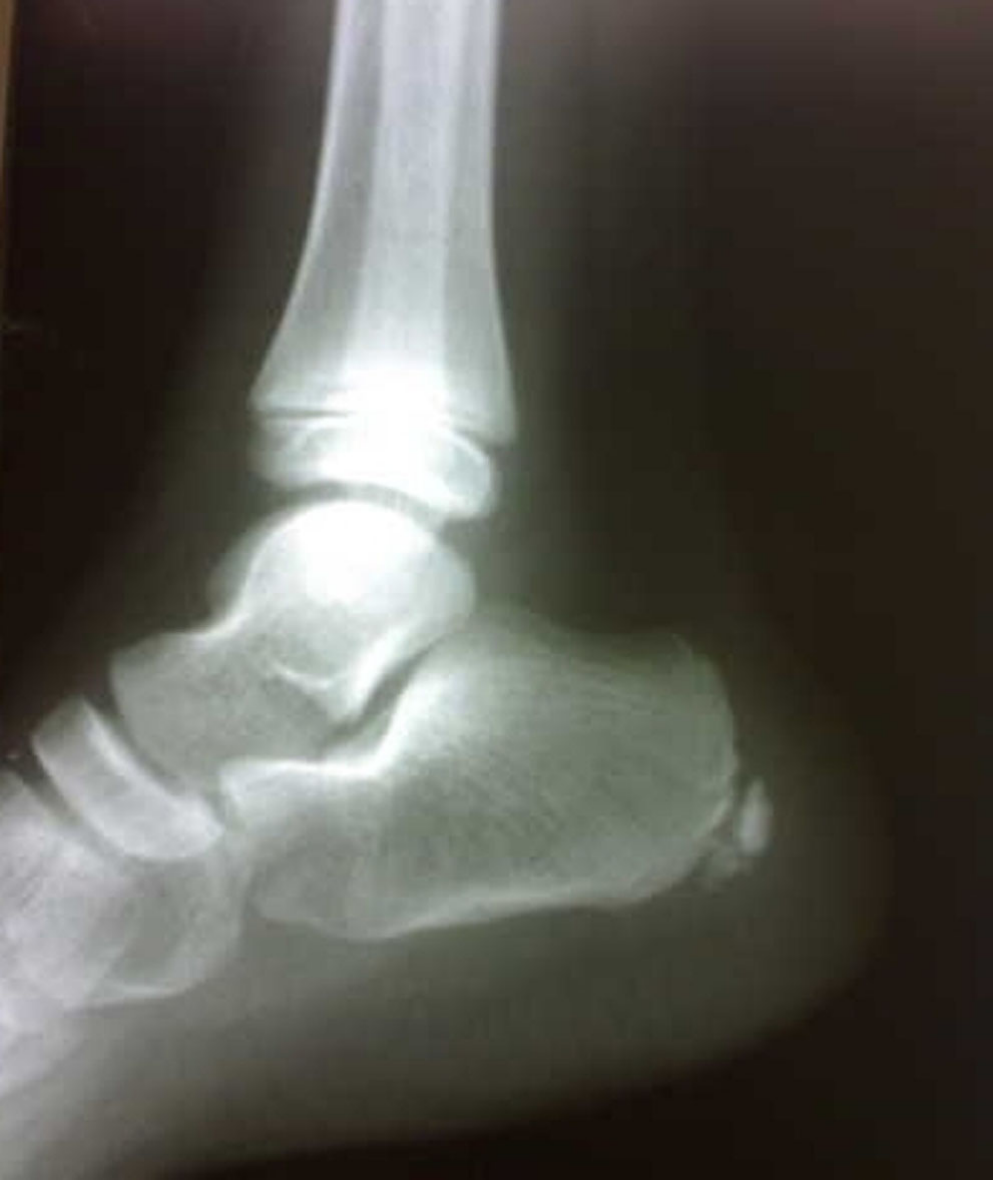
Lateral radiograph of the right foot. Note the sclerosis and fragmentation of the calcaneal apophysis.
